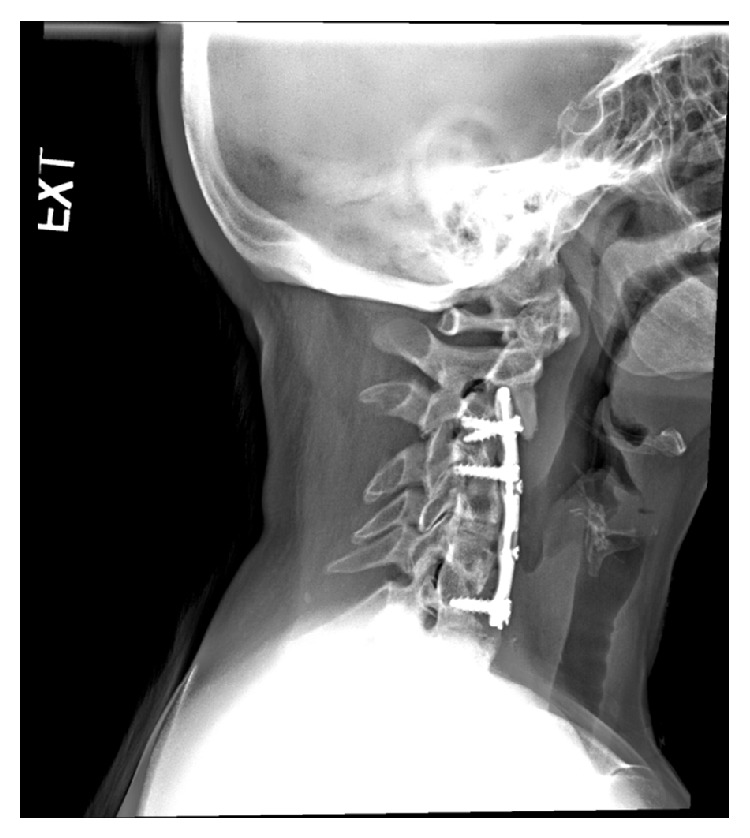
Plain radiograph, lateral view, of the cervical spine demonstrates air that appears to be communicating with plate.
